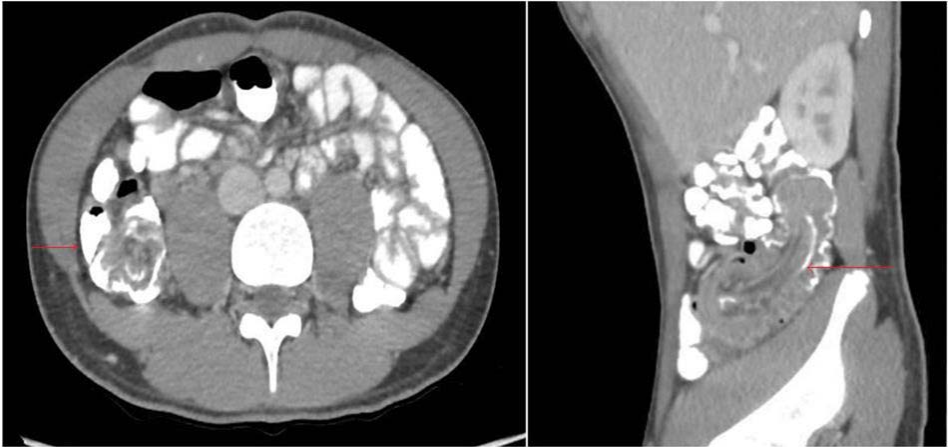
CT Abdomen (axial and sagittal plane) showing ileocolic intussusception extending for 10 cm with a soft tissue lesion at the tip of the intussusceptum concerning underlying lead point with reactive mesenteric lymph nodes.
